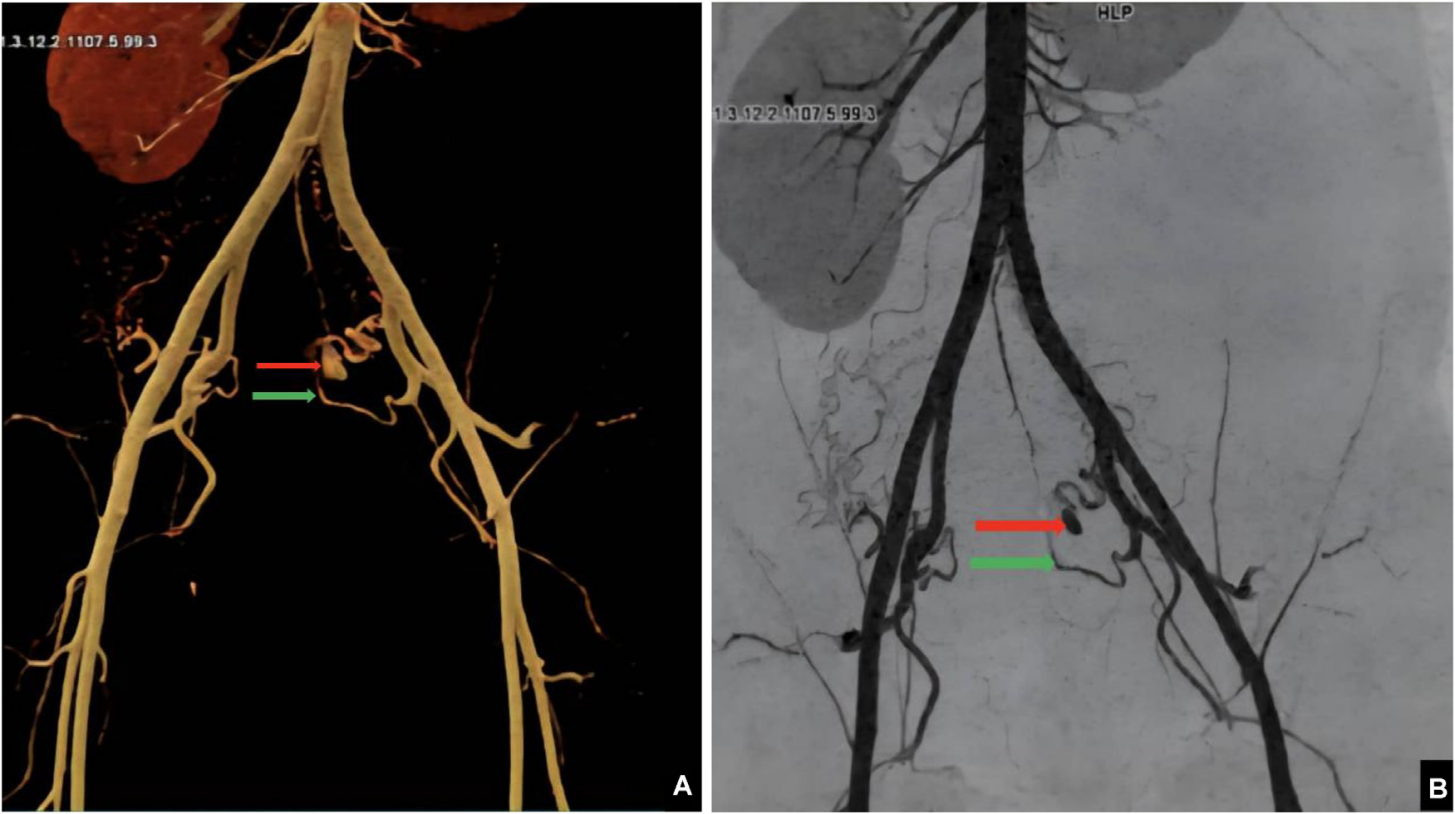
(A) Volume-rendered (VR) and (B) CT angiography image demonstrating a pseudoaneurysm (red arrow) originating from the left uterine artery (green arrow).
